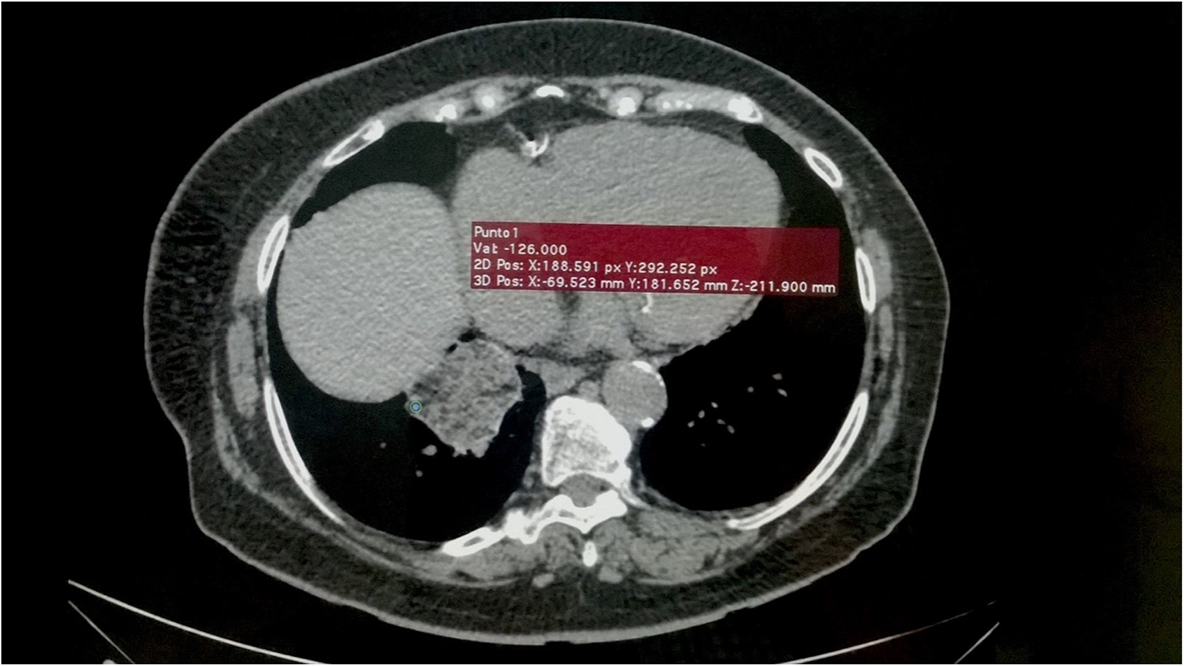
Thoracic CT at the first medical consultation
